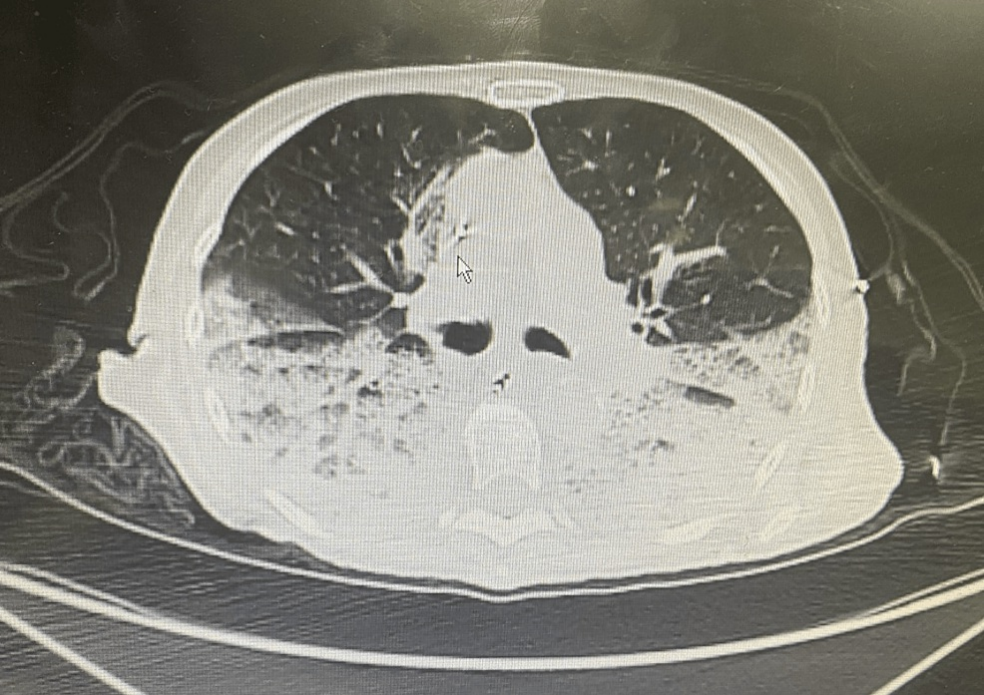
Computed Tomography of the Chest With Evidence of Bilateral Ground Glass Opacities
